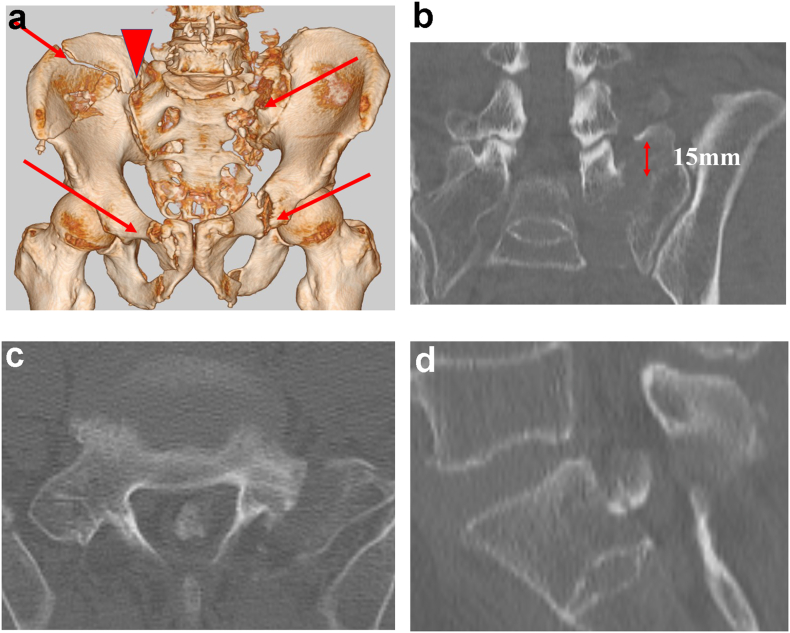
Preoperative computed tomography scan a: Arrows indicate fracture sites; arrowhead shows the sacroiliac joint dissociation. b: Sacral fracture with 15-mm vertical dislocation. c, d: A large free bone fragment in the spinal canal. (c, Axial view; d, sagittal view).
